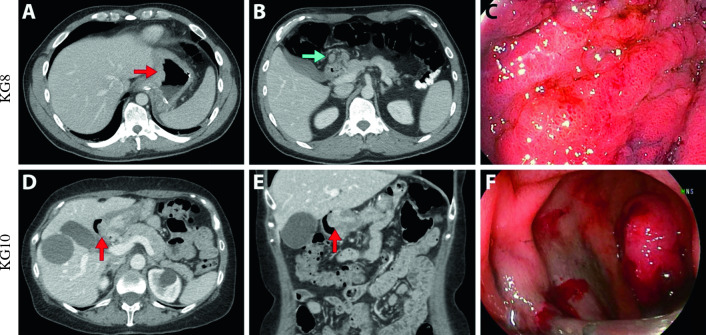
Patient-derived gastric cancers *KG8* and *KG10*. A Axial computerized tomography (CT) images of gastric cardia thickening. B Axial CT images with enlarged perihepatic lymph nodes. C Endoscopic images showing circumferential friable tumor extending from cardia to antrum consistent with linitis plastica. D Axial and E coronal CT images demonstrating ~3 cm polypoid mass at pylorus. F Endoscopic images demonstrating an obstructing mass. Red arrow: tumor, blue arrow: enlarged lymph node
